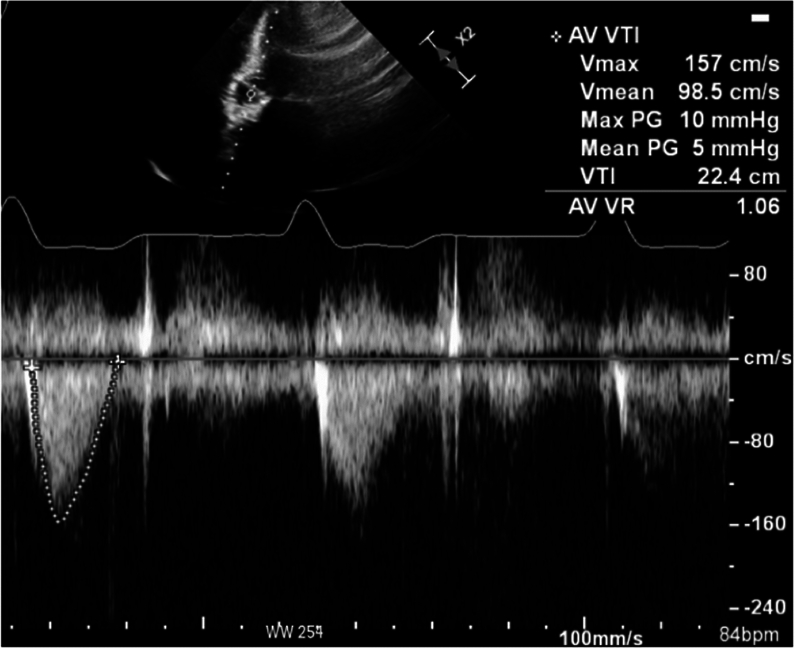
Continuous Doppler showed no significant LVOT obstruction. The peak and mean LVOT gradient were 10 mmHg and 5 mmHg, respectively
